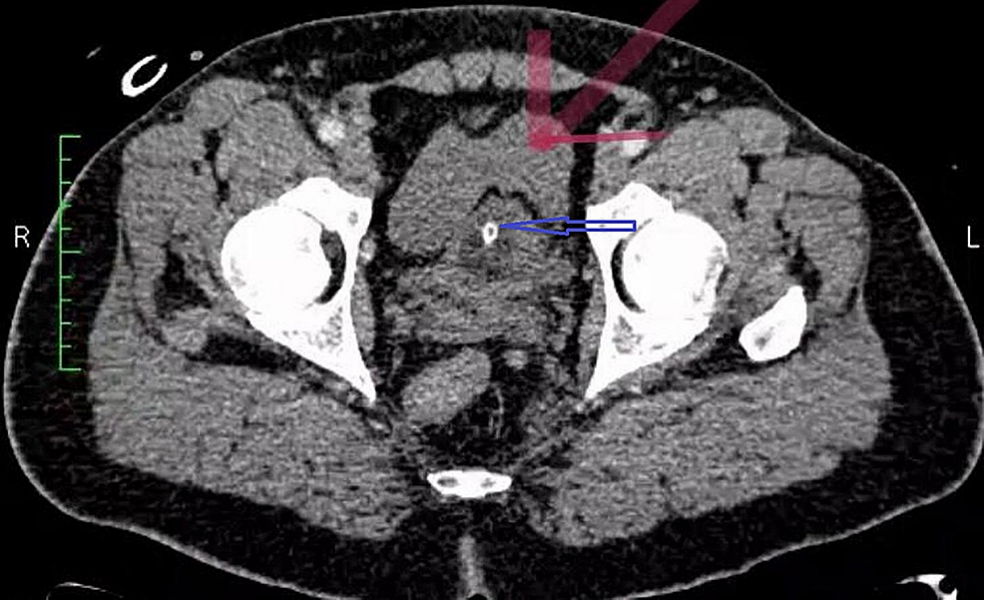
CT scan of abdomen and pelvis with intravenous contrast showing free fluid in the pelvis (red arrow) with urinary catheter in situ (blue arrow)
